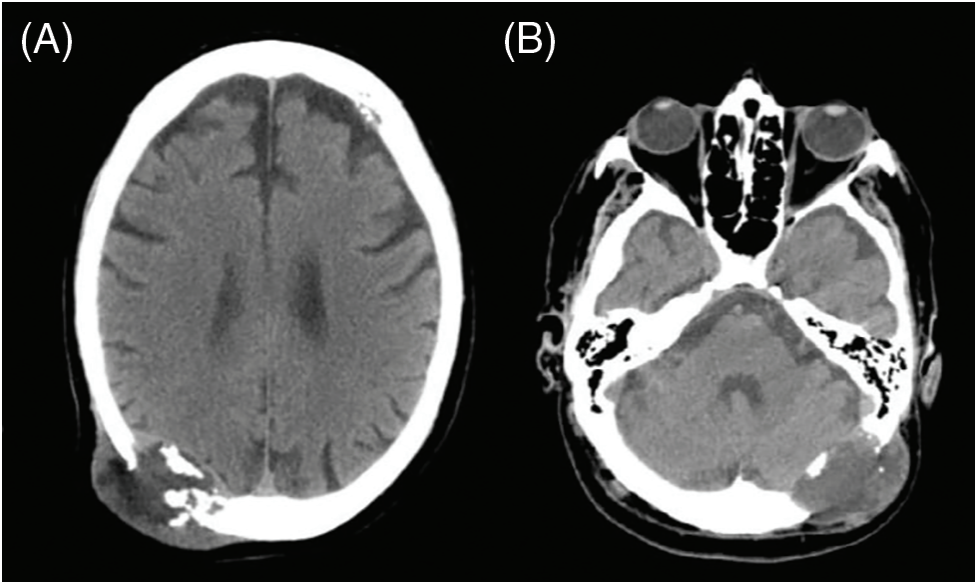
Head CT scan revealed extensive osteolytic lesions, the largest in the right parietal (A) and left occipital (B) regions.
